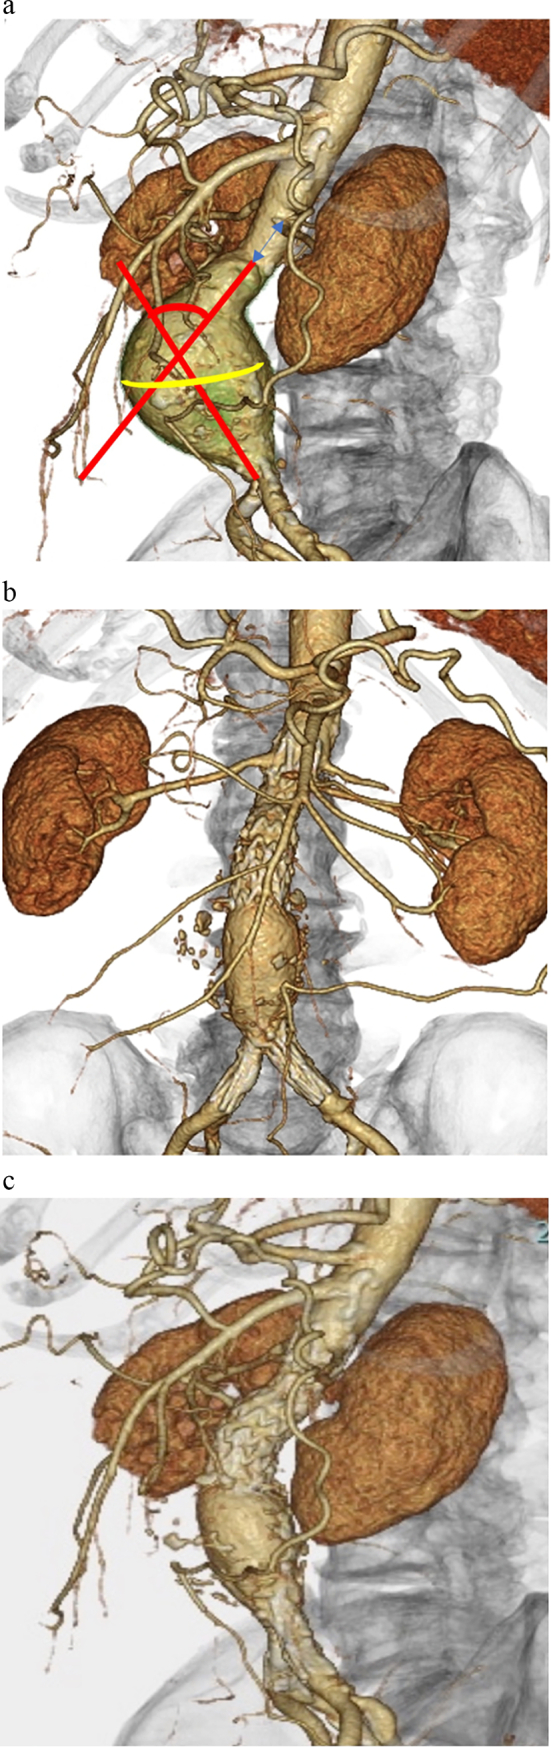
(A) Preoperative computed tomography (CT) shows an abdominal aortic aneurysm measuring 58 mm in diameter, with a proximal neck 20 mm in diameter and 15.6 mm in length. The proximal neck angulation is 60° in the anteroposterior direction. (B) (C) Postoperative CT shows a 25-mm overlap between the Endurant aortic extension cuff and main body.
